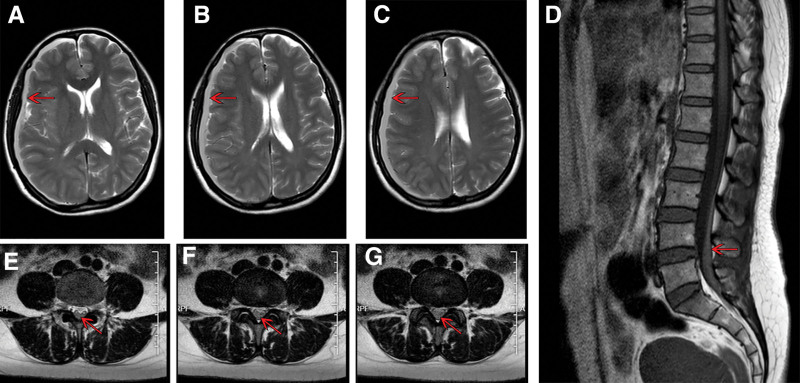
Lumbar spine and cranial MRI of the patient obtained on the day of admission. (A–C) Chronic subdural hematoma. (D–G) spinal subdural hematoma. The red arrow indicates a hematoma. MRI = magnetic resonance imaging.
